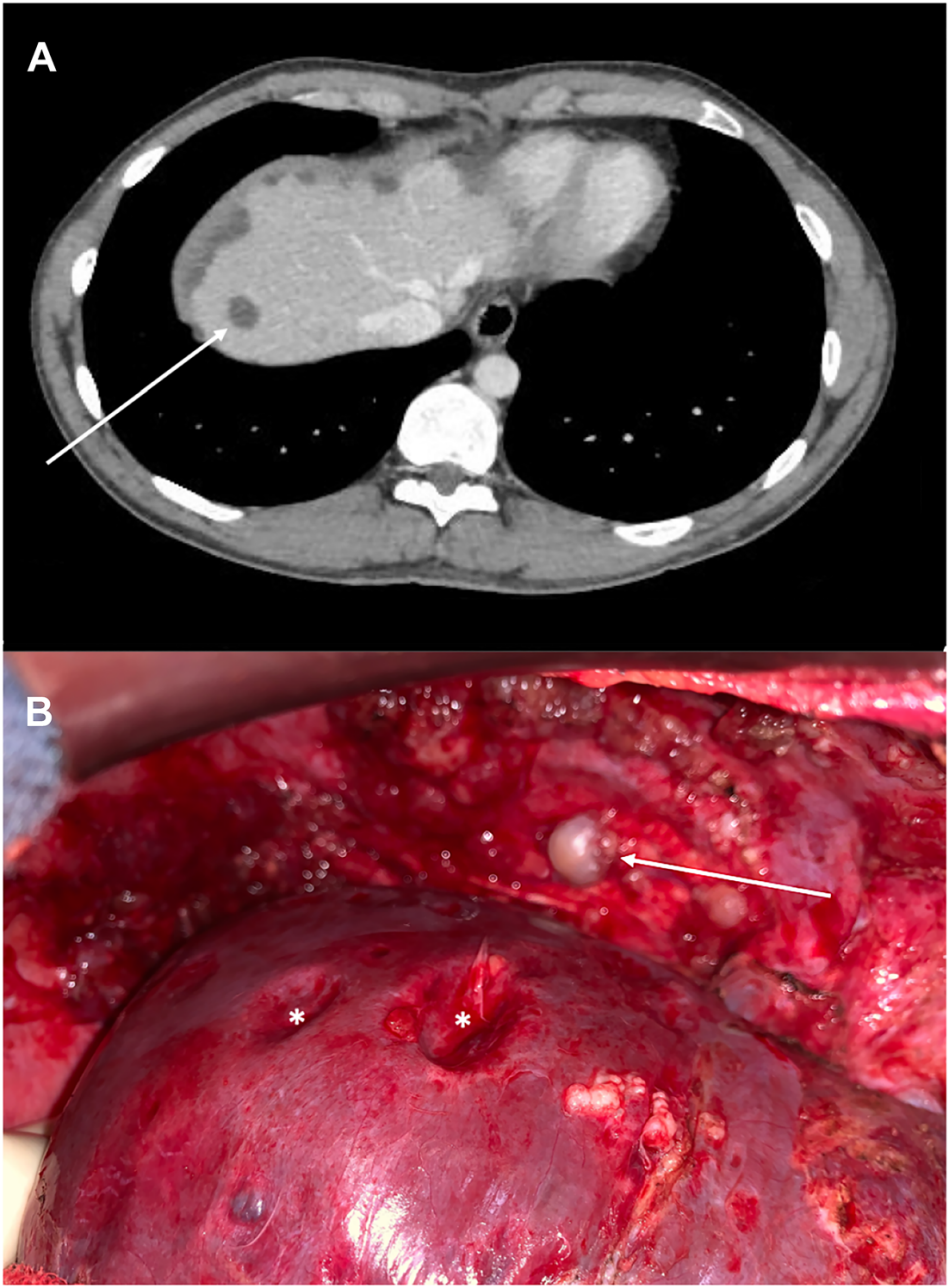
CT-scan (A) revealed hypodense liver lesions (arrow) at the diagnosis of left colon adenocarcinoma with synchronous peritoneal metastases. Explorative laparotomy (B) highlighted diaphragmatic peritoneal metastases (arrow) exerting pressure on the liver surface, called «scalloping» (star).
